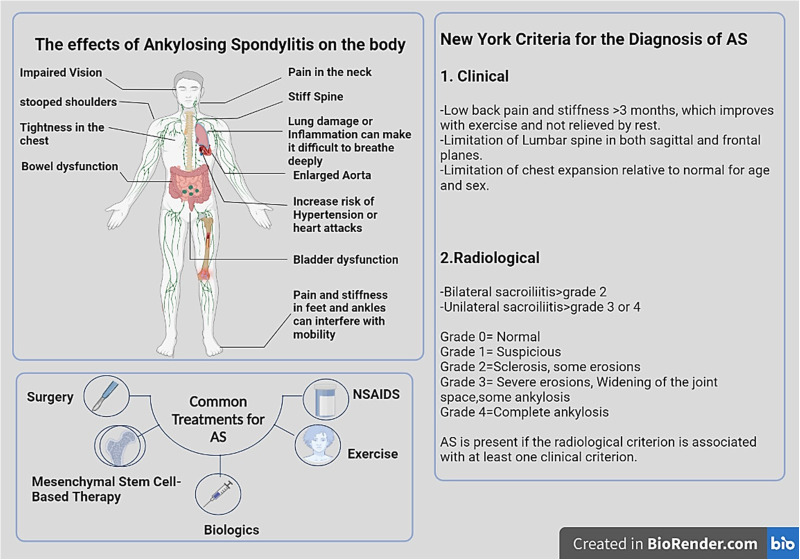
The physical manifestations of AS and a guide to diagnose and treatment. In AS, inflammation mostly affects axial joints, entheses, and extra-articular systems, including the uveal tract, digestive tract, mucocutaneous tissue, and heart. HLA-B27 is closely linked to spondyloarthropathies, particularly AS. In order to diagnose AS, at least one clinical indicator and one radiologic indicator are necessary. Bone scanning and magnetic resonance imaging (MRI) may help in the early detection of inflammation of the axial skeleton. Effective therapies for AS include local or systemic corticosteroid therapy, exercise, NSAIDs, DMARDs, methotrexate, azathioprine, anti-IL-17A monoclonal antibodies, TNF- antagonists, and MSCs therapy. Image was created with BioRender.com.
